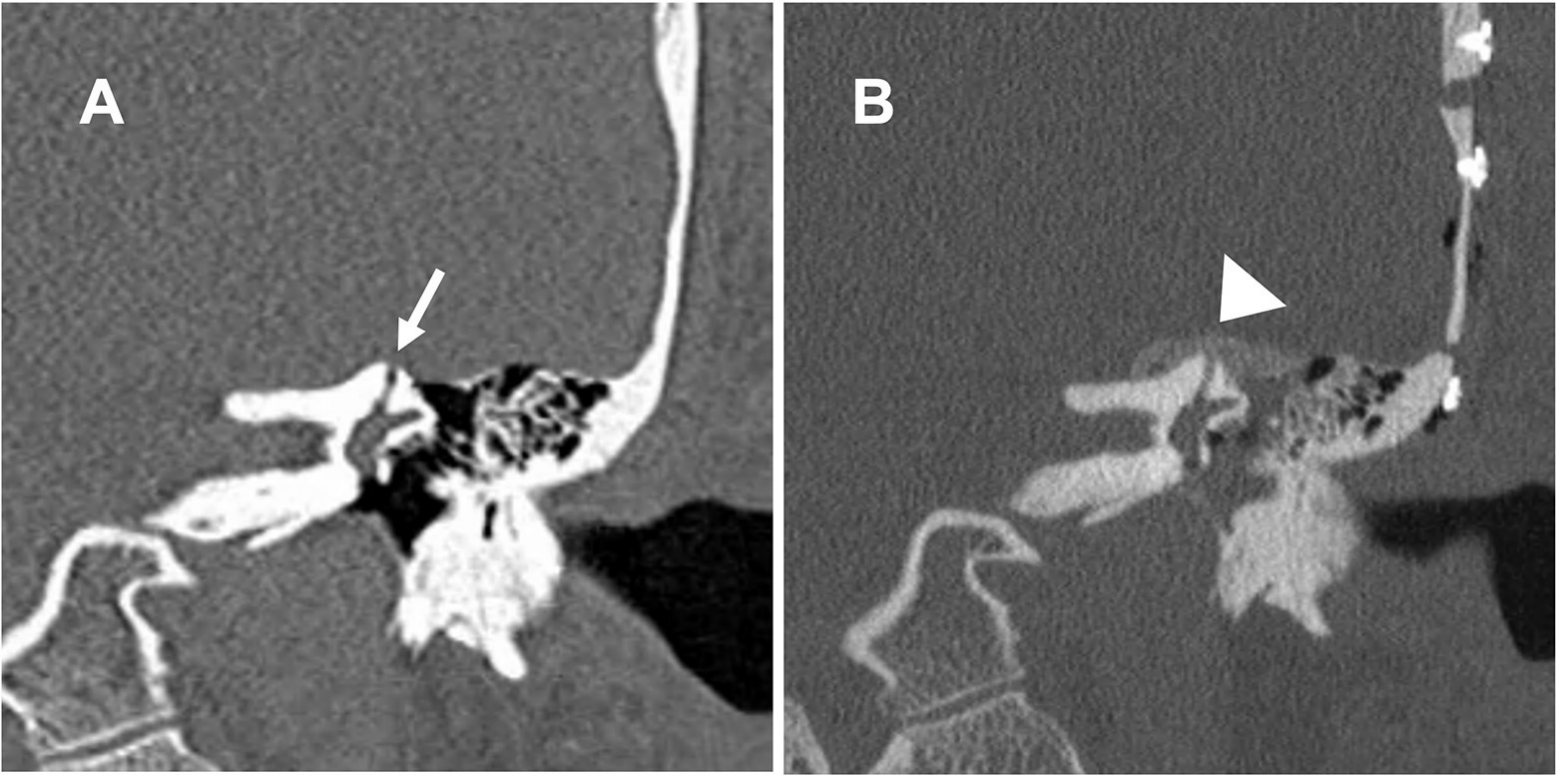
Coronal cut of a high-resolution CT scan of the left temporal bone. (A) The dehiscence of the left superior canal (arrow). (B) The bone dust plugging the dehiscence and covering the superior canal (arrowhead).
